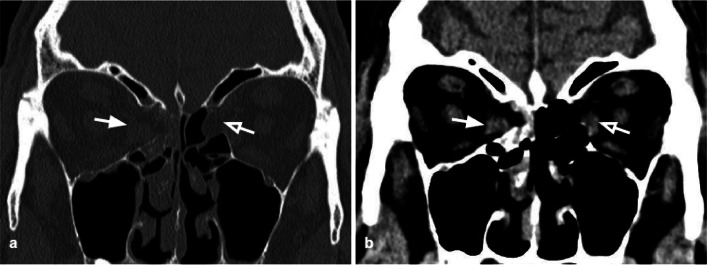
Coronal CT reconstruction with bone (a) and soft tissues (b) algorithms in a patient with a current fracture of the right orbital medial wall (white arrow) and a previous fracture of the contralateral orbital medial wall (empty arrow). a Huge bone defect of the right lamina papyracea (white asterisk). On the left side, discontinuation of the lamina papyracea caused by a previous fracture is visible, as well as the herniation of the intraorbital fat tissue into the ethmoidal cells (empty arrow). b The medial rectus is thickened and partially displaced through the bone defect (arrow). The left medial rectus muscle shows regular site and morphology (empty arrow)
